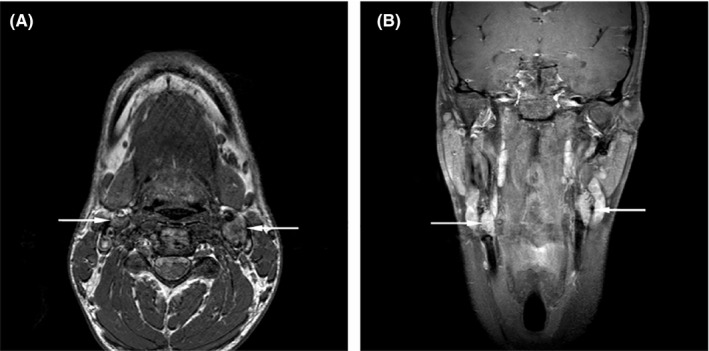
Magnetic resonance imaging (T1 weighting) of a 31‐year‐old male (family member V.4) diagnosed with bilateral, carotid hnPGL shown in axial A, and coronal B, views (arrowed)
